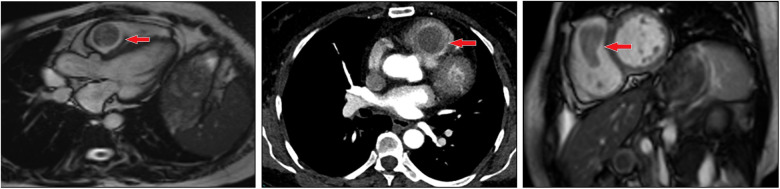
Imaging of metastasizing leiomyoma. Enhanced MRI revealed soft tissue signal mass shadows in the outflow tract of the right ventricle.
